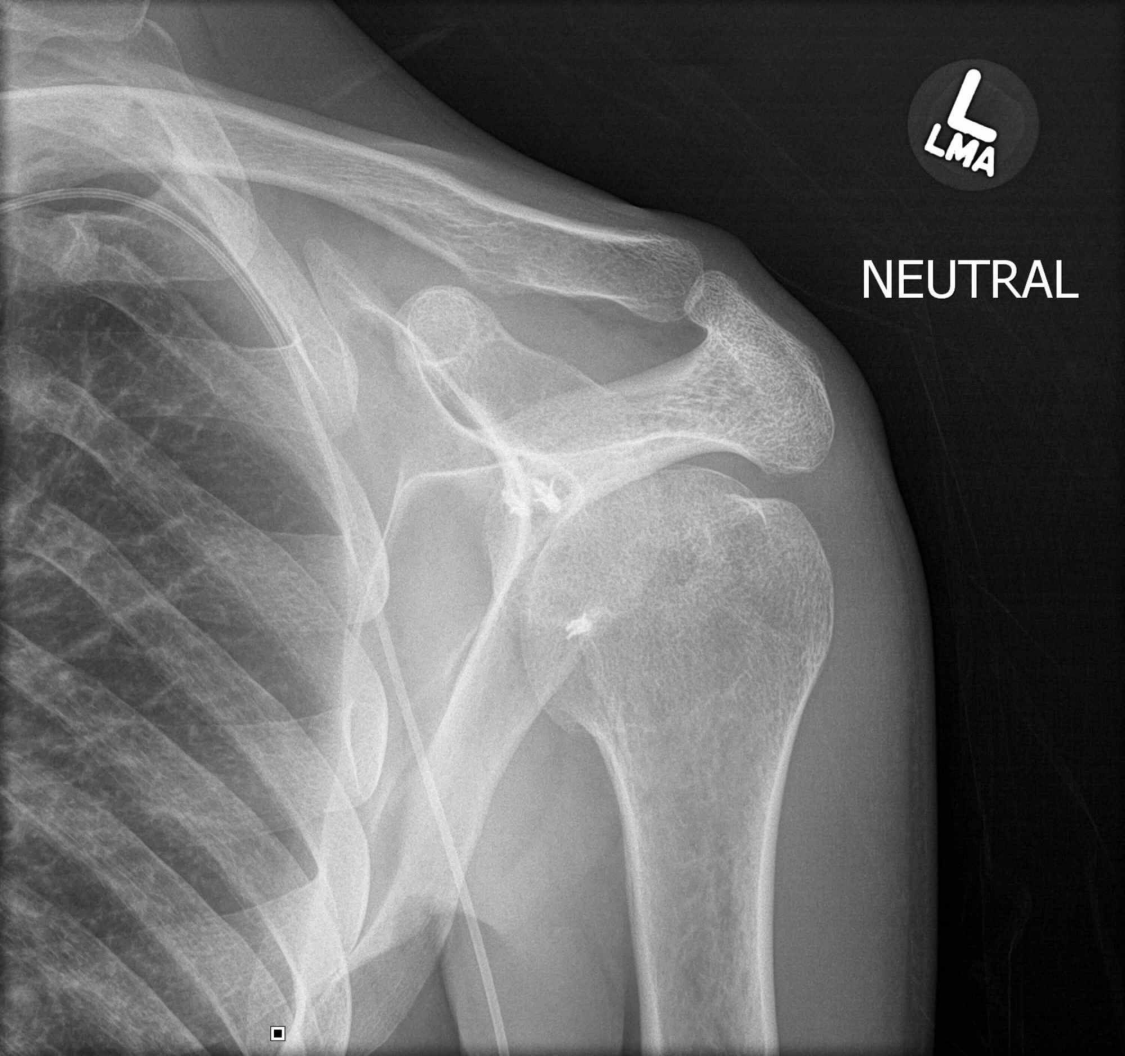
AP internal rotation radiograph of the shoulder showing posteroinferior subluxation of the humeral head AP: anteroposterior
